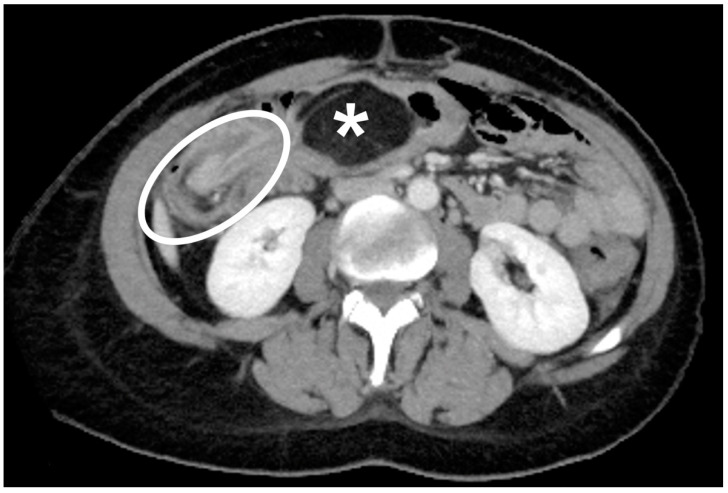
Axial CT scan of the abdomen in portal/venous phase. A rounded, smoothly outlined and sharply demarcated abdominal mass, having fat attenuation mass with fat attenuation (asterisk) is observed. A circumferential thickening in ileocolic intussusception caused by the lipoma can be seen (white circle).
